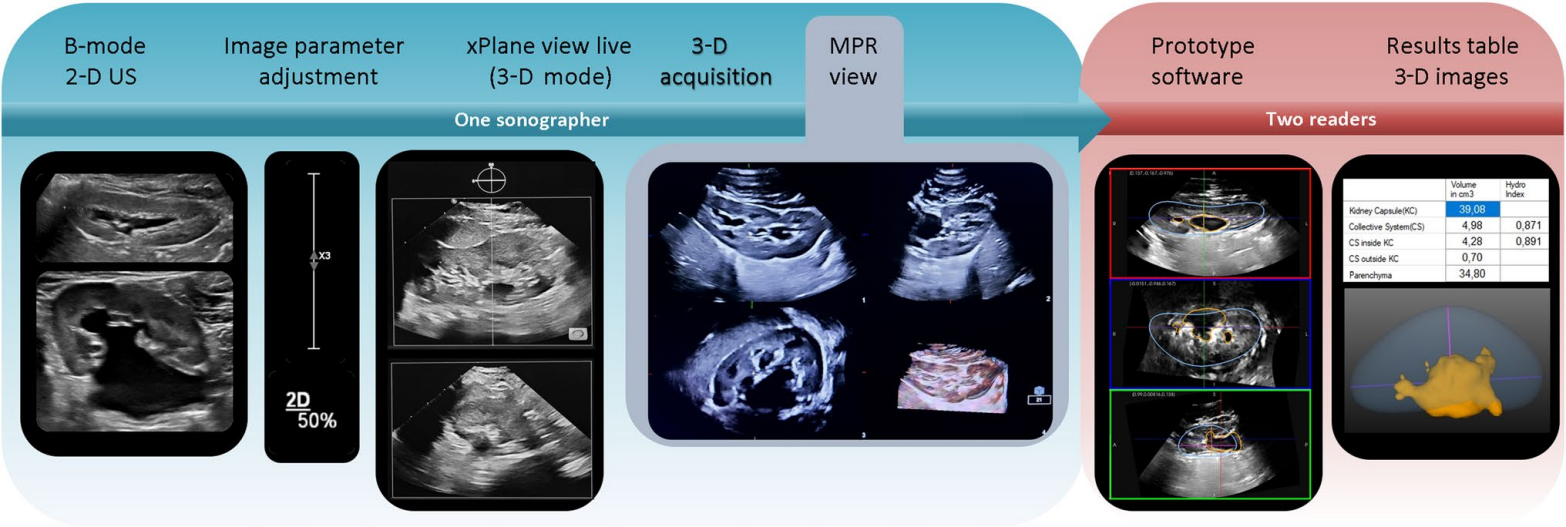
Workflow of the study. Image parameters were adjusted based on the conventional 2-dimensional (D) B-mode ultrasound. Afterward, the 3-D mode display was used to show live side-by-side-image including a sagittal and axial view of the kidney at the same time. After 3-D volume acquisition the multiplanar reformatted (MPR) view highlights the structure in three orthogonal planes and a volume-rendered image. The segmentation was performed independently by two radiologists on a separate workstation
